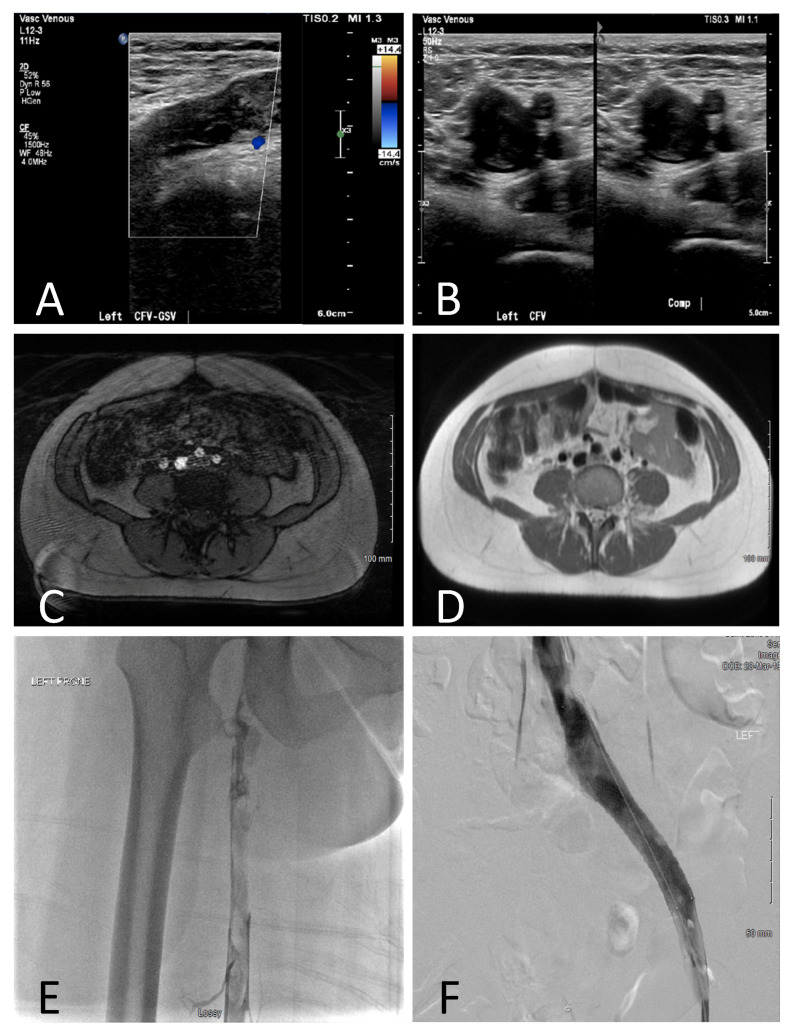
Diagnostic Imaging from Patient 1. Duplex/Doppler ultrasound demonstrating occlusive thrombus in the CFV-GSV (A). Coronal T1-weighted (B) and axial T2-weighed MRA/V (C) demonstrating compression of the LCIV between the spine and RCIA, with extensive thrombus (arrows). IVUS demonstrating occlusive thrombus (D). Venography before (E) and after (F) stent placement postpartum.
